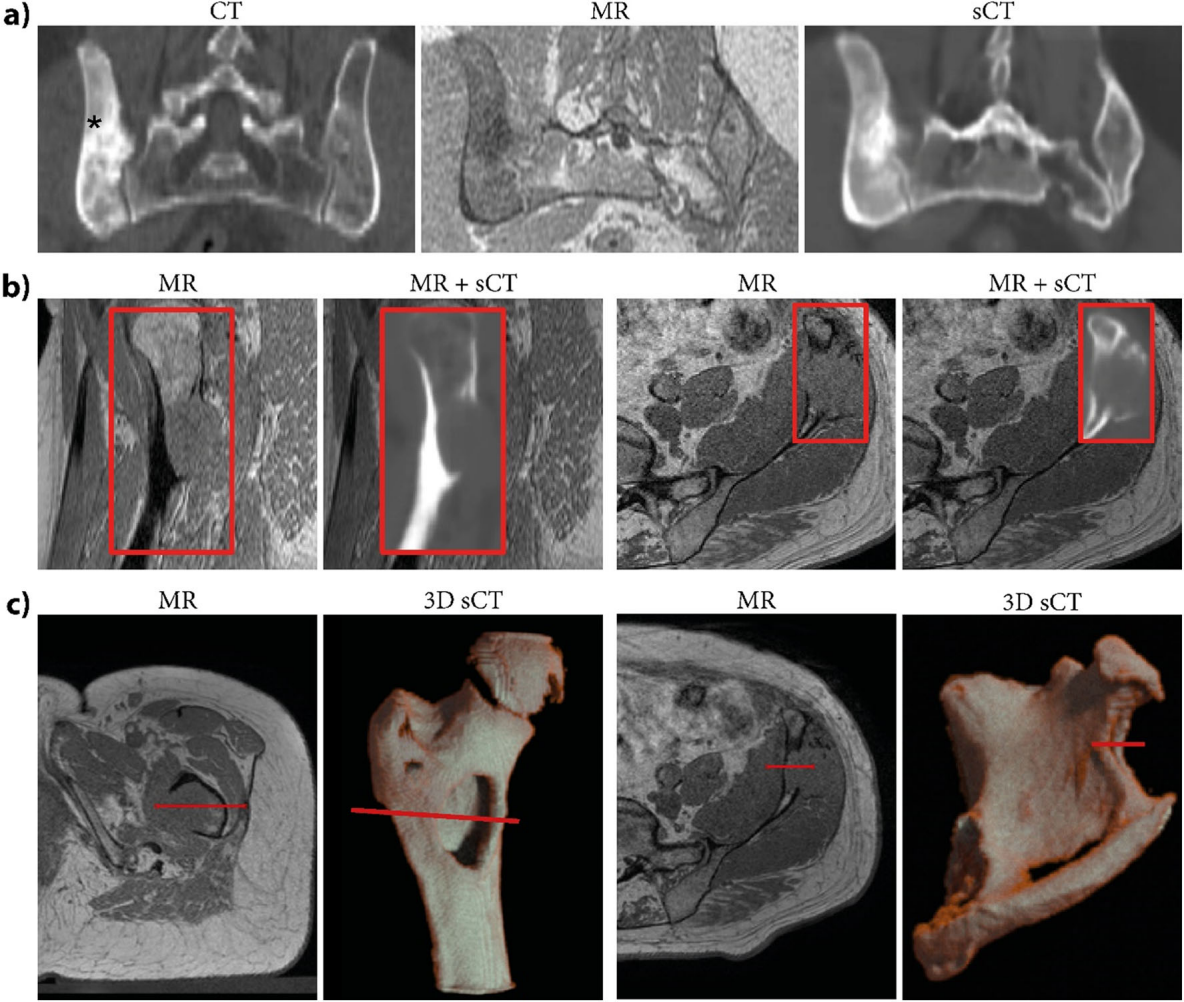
a Pretreatment CT images in the pelvis with conventional patient positioning and MR and intrinsically aligned synthetic CT (sCT) images with unconventional patient positioning for the HIFU treatment. Lesion is indicated by *. Since sCT scans are intrinsically registered with MR, they are able to provide bone cortex depiction with the patient in treatment position. b MR and a fused visualization of MR and sCT images depicting the cortical bone distribution, obtained for two patients with osteolytic lesions in the femur (left) and in the pelvic iliac crest (right). MR and sCT are inherently registered, allowing straightforward identification of soft tissues and bone. c MR images from transverse slices of two patients with lesions in the femur and pelvis and corresponding 3D bone renderings that provide an overview of the bone with the lesion. The bone renderings were obtained by thresholding the sCT at 150 Hounsfield units within the bone mask (created in MeVisLab v3.2, MeVis Medical Solutions AG). The red line identifies the same location in MR images and 3D bone renderings
